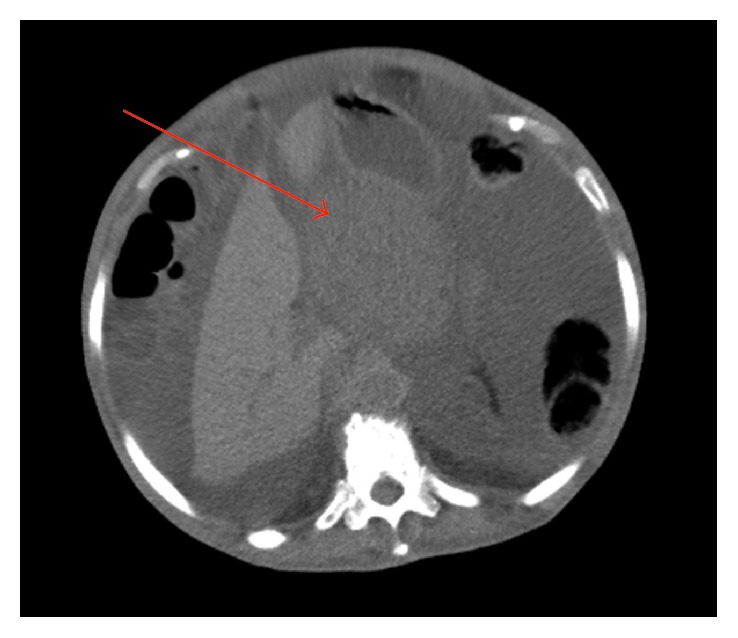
CT scan showing a lobulated mass in front of the pancreas measuring 8.5 × 9.5 cm (arrow), signs of chronic liver disease, and high-volume ascites.
